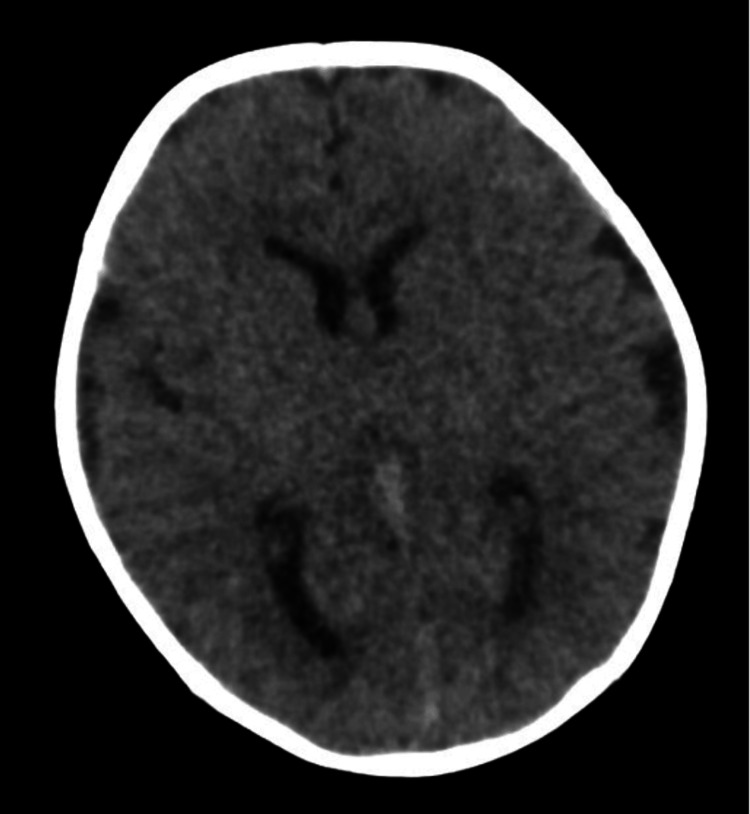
CT head without contrast showing no acute intracranial abnormalities. CT: computed tomography
